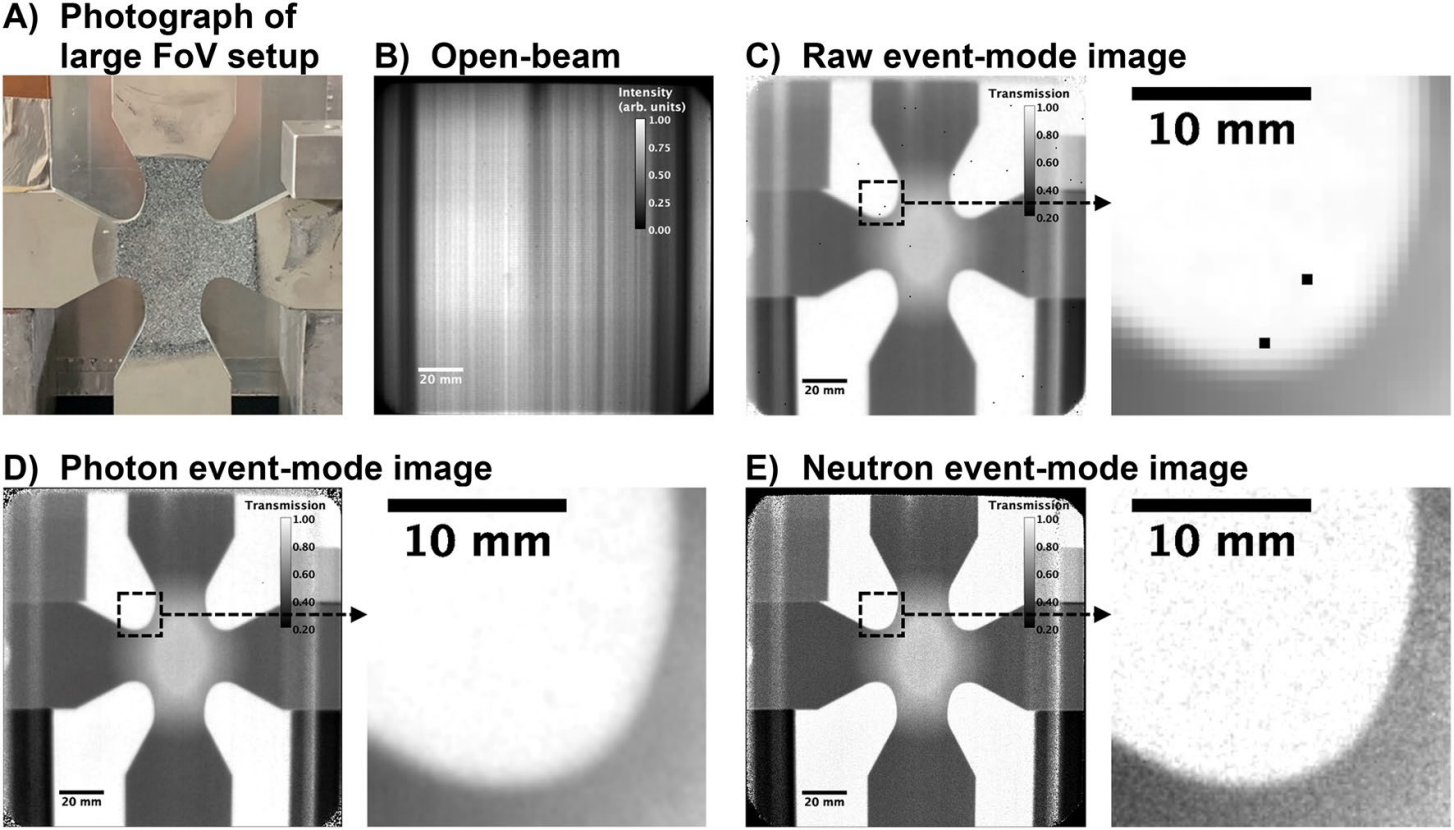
Photograph of various objects positioned at 10 cm distance to the scintillator in (A) (steel cross in the center, lead bricks on the bottom left and right, copper sheets on the top left and aluminum cube on the top right). Open-beam using CoM neutron events at sub-pixel resolution of ¼ pixel-pitch in (B). Normalized radiographs, including an enlarged section of ~ 2 × 2 cm^2^, produced from: raw event data using the native resolution of the chip in (C); CoM photon events at sub-pixel resolution of ½ pixel-pitch in (D); CoM neutron events at sub-pixel resolution of ¼ pixel-pitch in (E).
